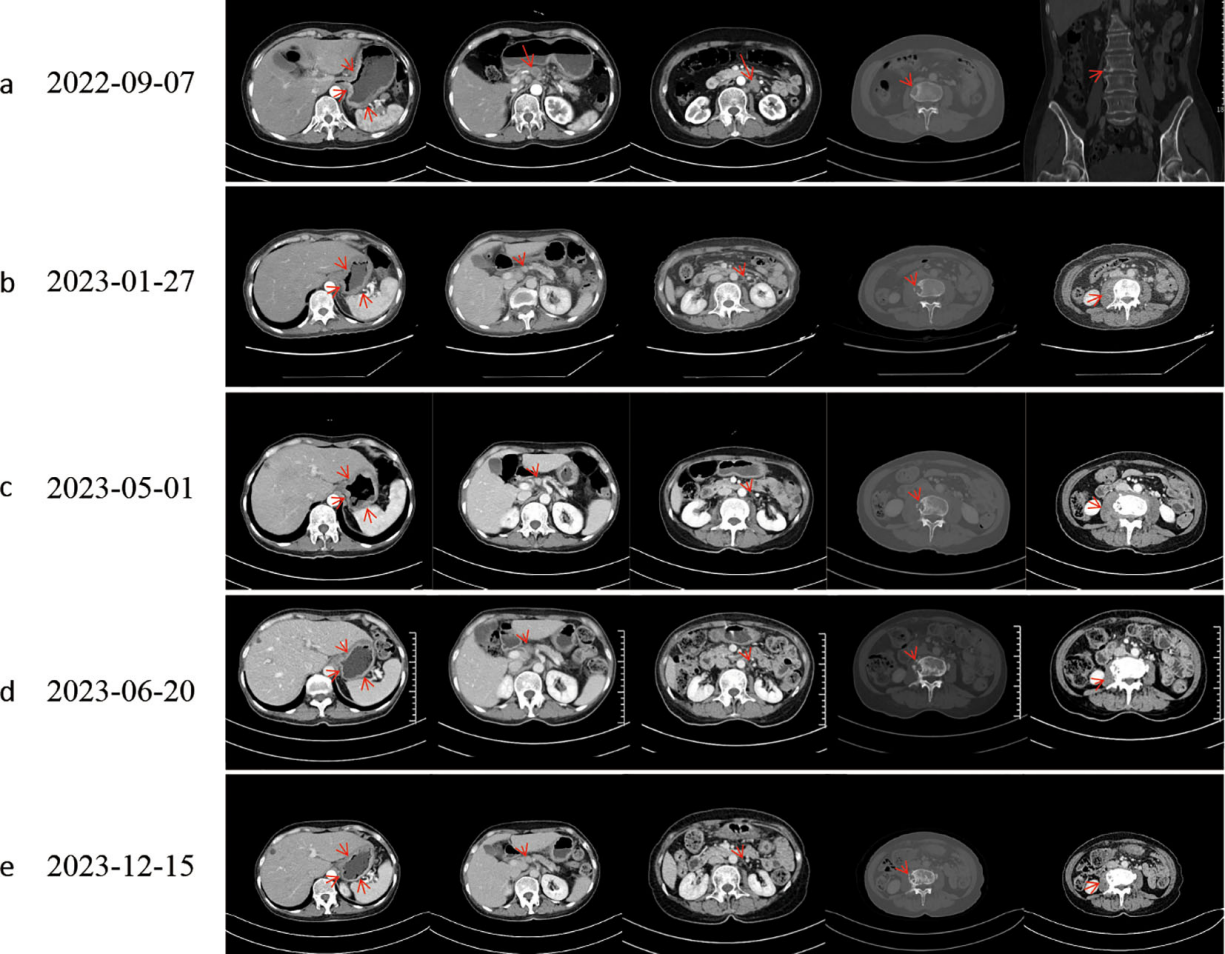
Computed tomography evaluation. Following effective therapy, the patient exhibited decreased gastric wall thickness, reduced osteolytic destruction of bone metastases, increased osteoblastic changes, and diminished perilesional soft-tissue components around osseous metastases. (a) Baseline. (b) Evaluation after progression of first-line chemotherapy. (c) Evaluation after progression of second-line chemotherapy; L3 vertebral metastasis showed progressive osteolytic destruction with an expanding perilesional soft-tissue mass. (d, e) The best response to targeted therapy was a partial response (PR).
